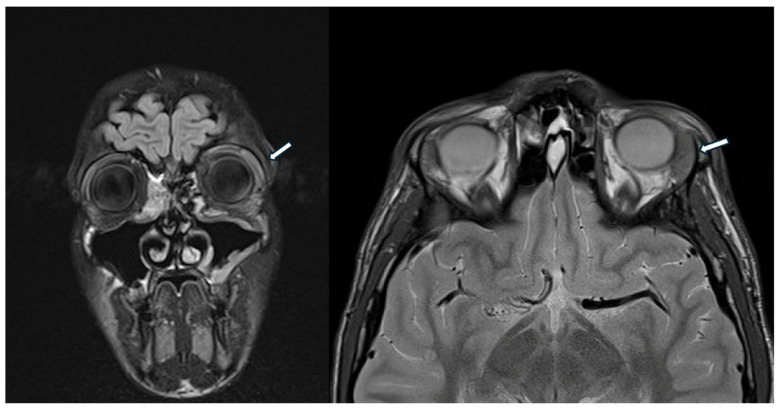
The head MRI shows enlarged left lacrimal gland (white arrow) and minimal peribulbar fluid.
